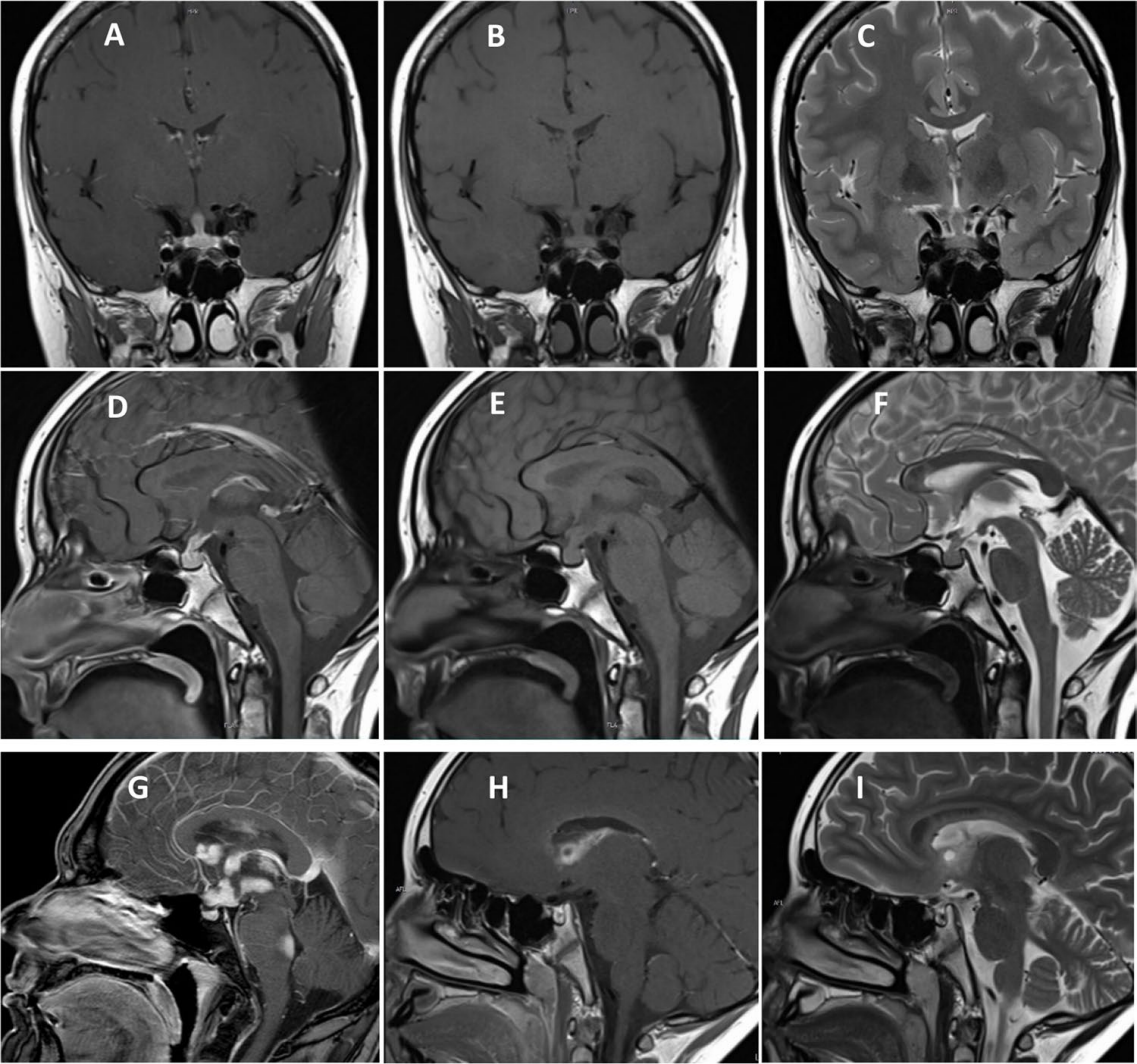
Typical magnetic resonance imaging of intracranial germinoma. Coronal (A–C) and sagittal (D–F) contrast-enhanced T1-weighted (A, D), T1-weighted (B, E), and T2-weighted (C, F) images shown a germinoma involving the pituitary stalk. Contrast enhanced T1-weighted sagittal image shows a disseminated germinoma (G). Contrast-enhanced T1-weighted and T2-weighted images show a solid germinoma with a cystic component located in the right lateral ventricle wall (H, I)
